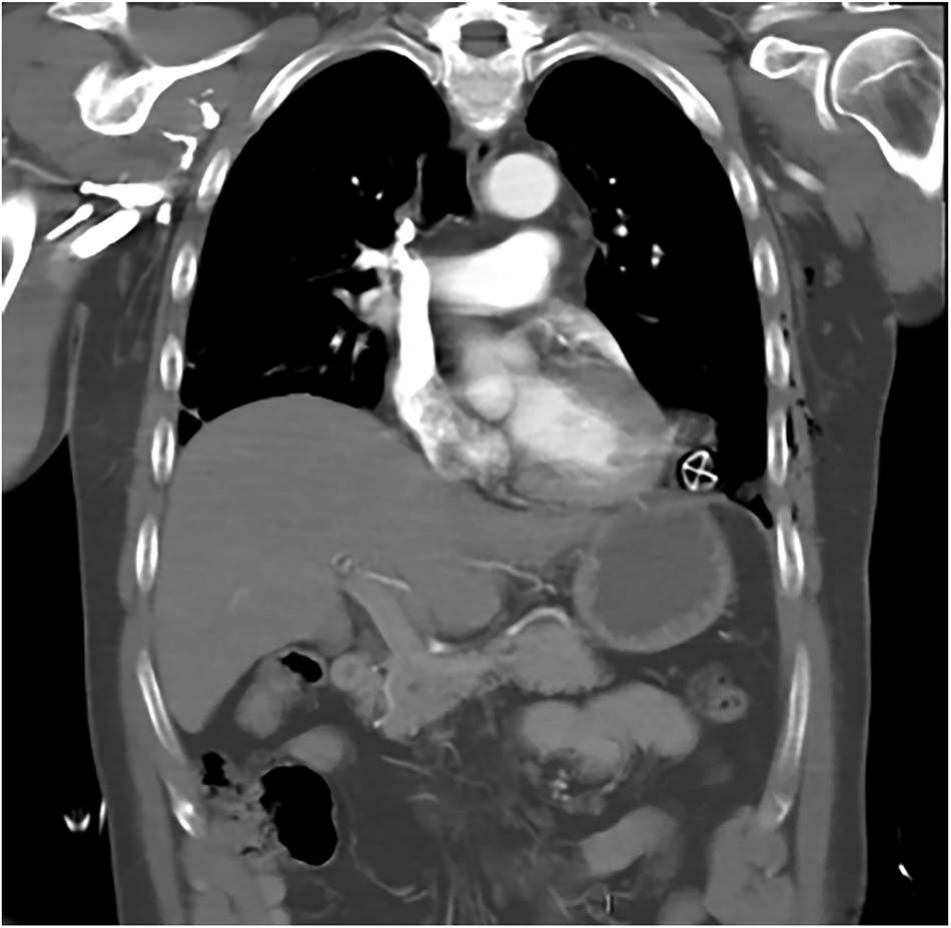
CT coronal image showing solar powered garden light proximity to diaphragm and cardiac border.
